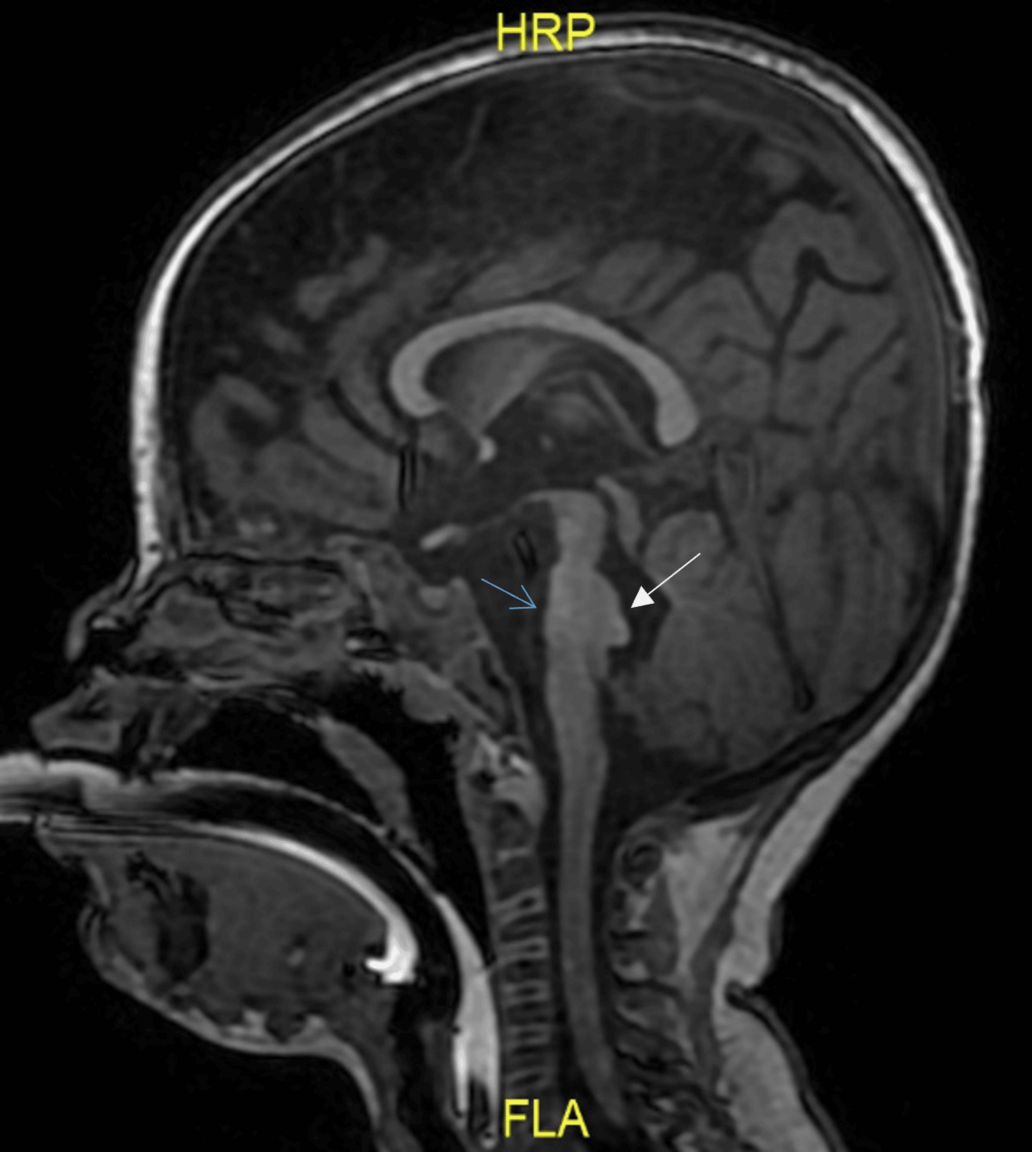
Sagittal T1-weighted image shows hypoplastic pons with flattening of the ventral surface (blue arrow) and dorsal projecting white matter cap (white arrow) into the fourth ventricle.
